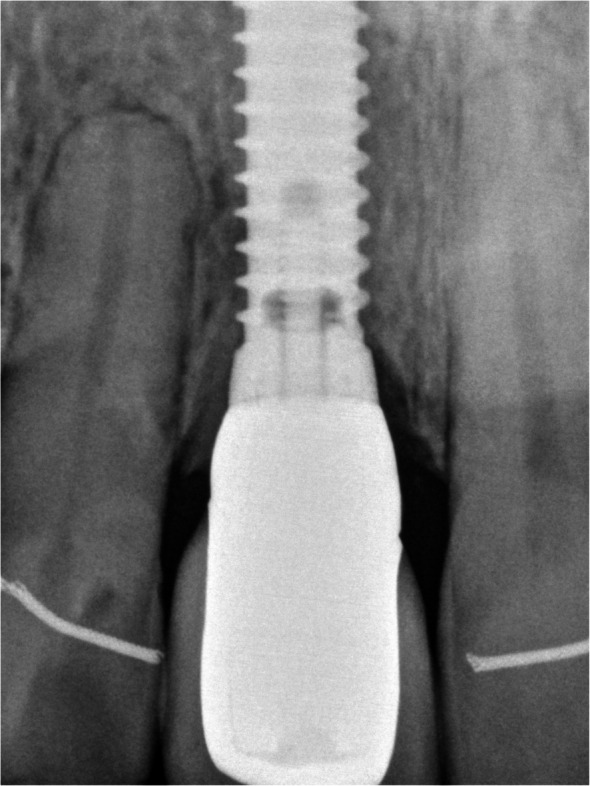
Postoperative x-ray after osseointegration
